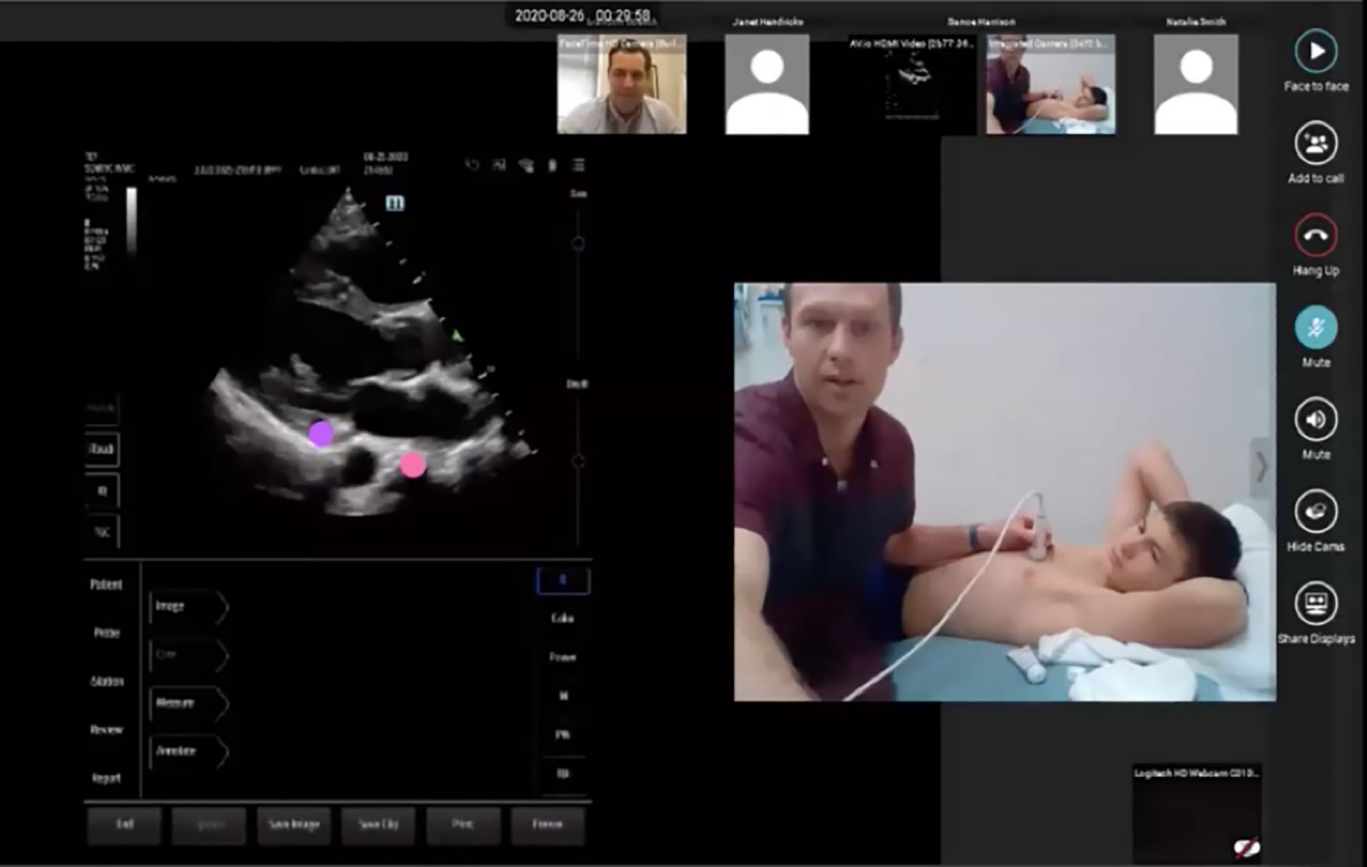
Tele-ultrasound hands-on scanning session. Live hands-on scanning sessions were conducted using tele-ultrasound software (Reacts by Philips & Innovative Imaging Technologies). The platform allowed simultaneous visualization of the learner’s hands position (right) and ultrasound screen (left) which allowed faculty to guide learners in image acquisition. Both faculty and learners could point to structures on the ultrasound screen using different colored pointers (left)
